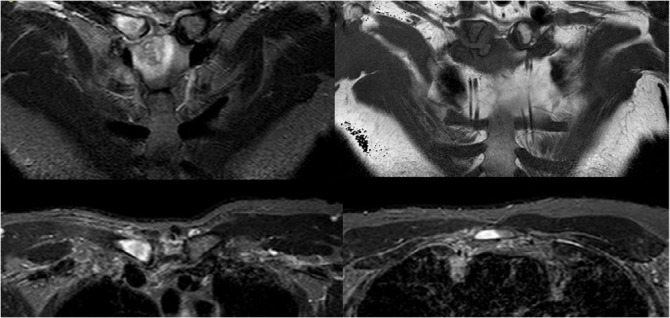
Magnetic resonance imaging showed mild synovial reaction and intra-articular effusion with prominent bone marrow oedema.
